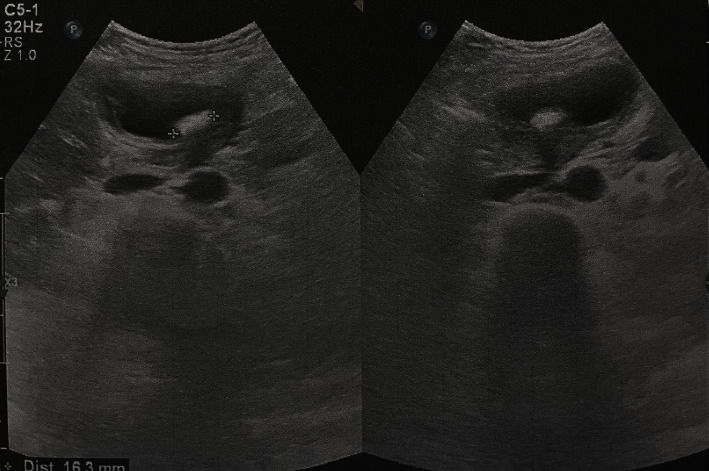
Ultrasound picture of the gallbladder showed an increased wall thickness and a single stone inside the cavity of the gallbladder.
